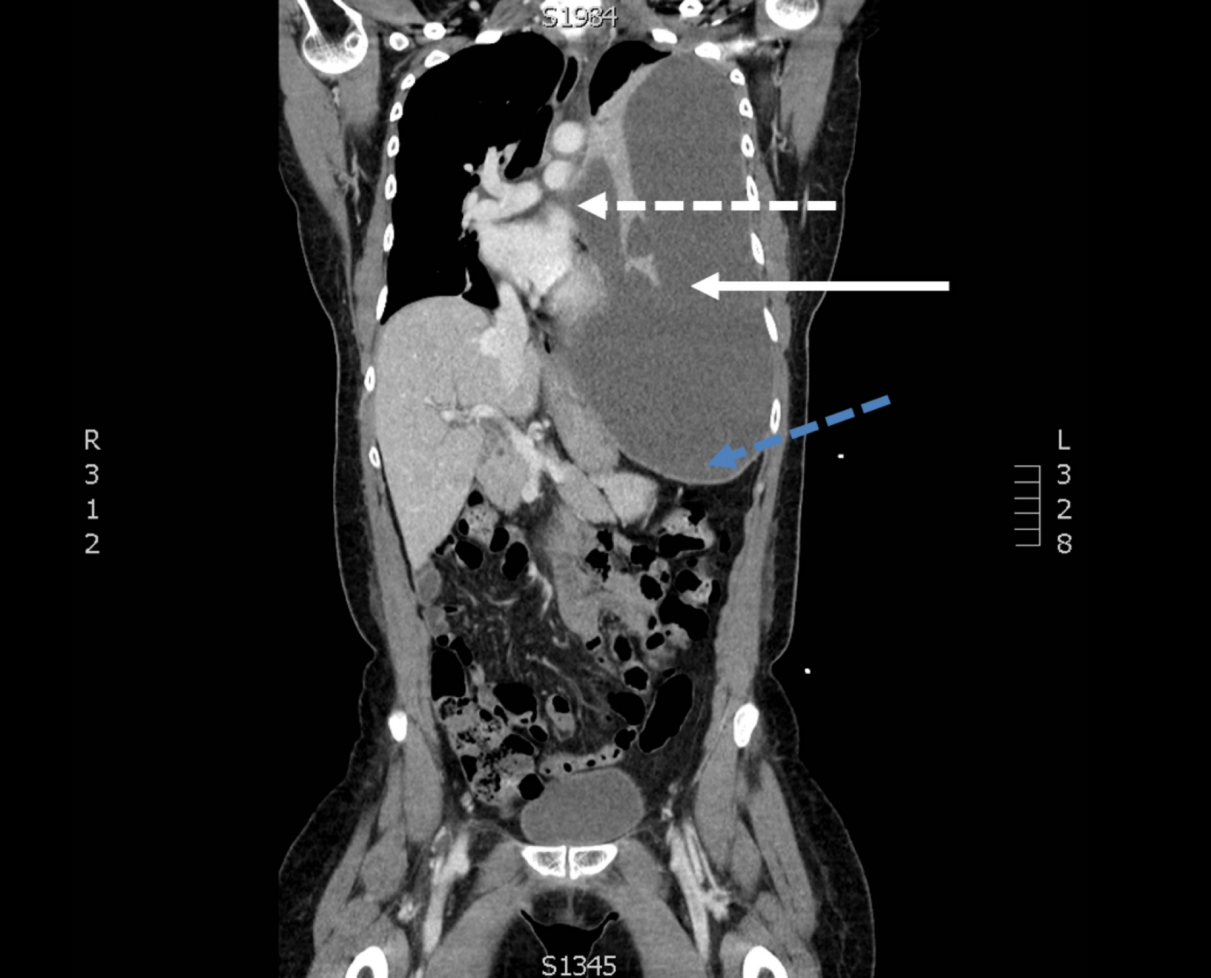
A coronal slice of a contrasted computed tomography of the chest, abdomen, and pelvis demonstrating a large left-sided pleural effusion (solid white line) with mass effect on the mediastinum (dashed white line) and left hemidiaphragm (dashed blue line)
